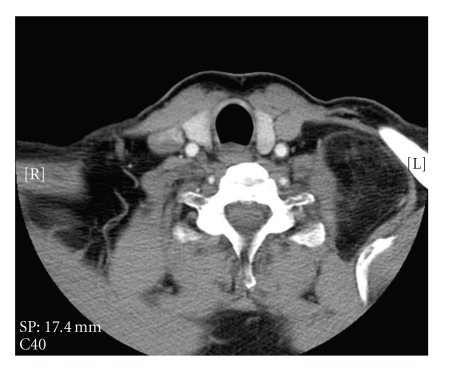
Axial CT scan of the neck showing extension of left supraclavicular mass posteriorly.
